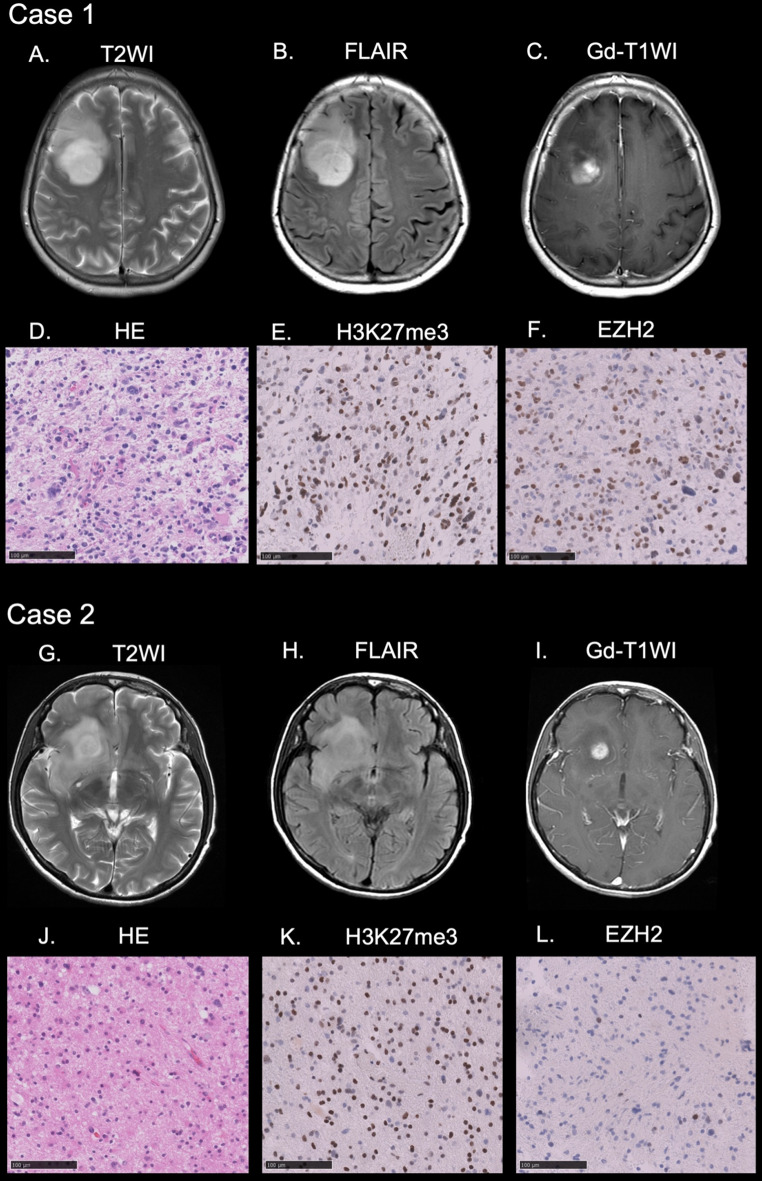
Case 1. MRI revealed a right frontal lobe tumor with hyperintensity on T2WI (A) and FLAIR (B). The tumor was partially enhancing on post-contrast T1WI (C). HE revealed a diffuse astrocytic tumor (D). IHC revealed positivity for H3K27me3 (E) and EZH2 (F). Case 2. MRI revealed a right frontal lobe and insular tumor with hyperintensity on T2WI (G) and FLAIR (H). The tumor was partially enhancing on post-contrast T1WI (I). HE revealed a diffuse astrocytic tumor (J). IHC uncovered positivity for H3K27me3 (K) and negativity for EZH2 (L)
